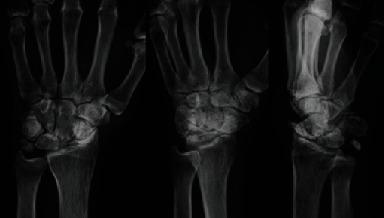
Plain X-ray findings show sharpening of the styloid process, narrowing between the radial scaphoid bones, and growth of the dorsal ridge.
